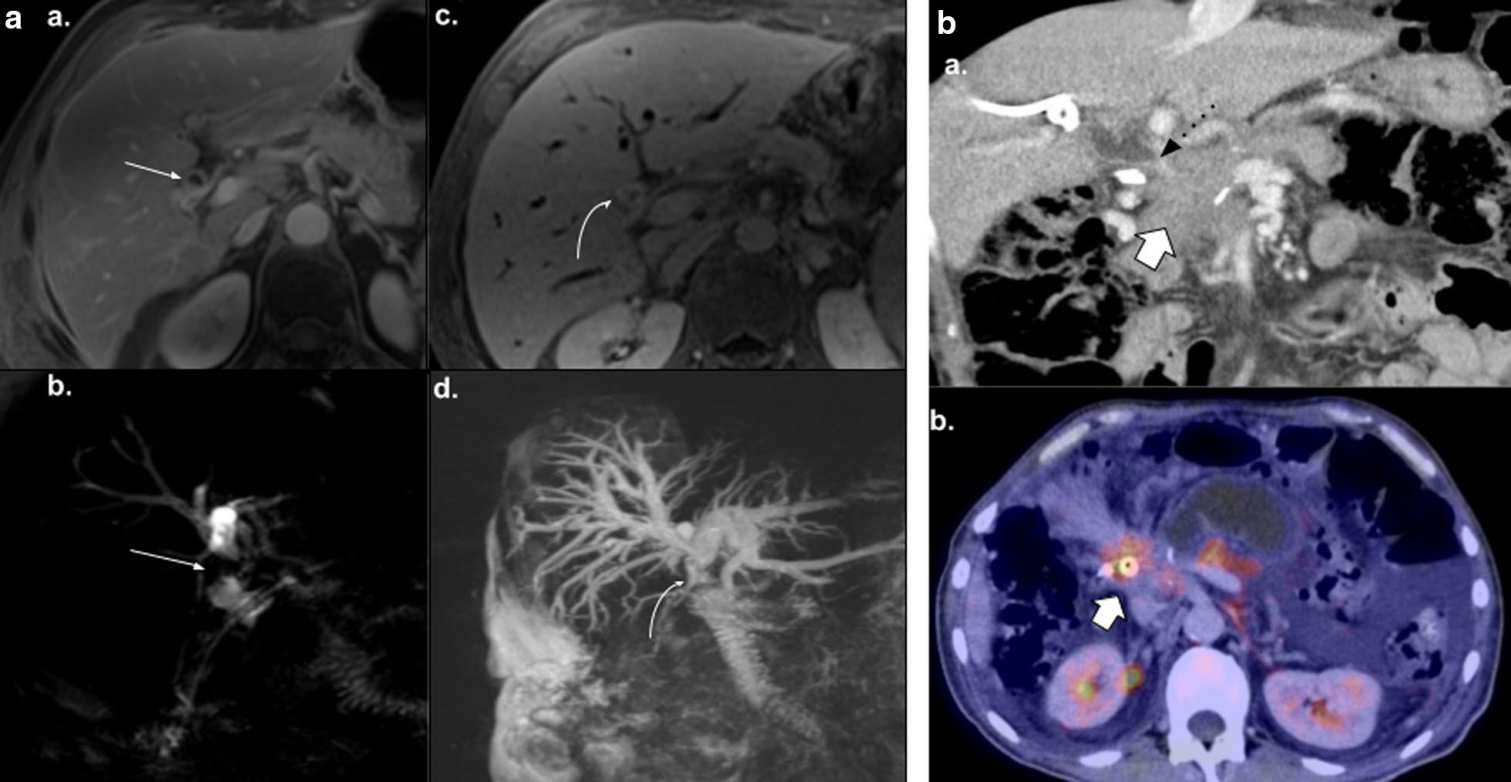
Hepaticojejunostomy stenosis. Two different patients (a) 67-year-old male patient with pancreaticoduodenectomy due to cholangiocarcinoma. All images below to normal postoperative control MRI. (a, b) one month after surgery (c, d) 7 months control. Note the caliber reduction in hepaticojejunostomy due to thickness of hepatic bile duct (curved arrows). Intraoperative cholangiography confirmed total hepaticojejunostomy stenosis (not shown) (b) 59-year-old male patient with abdominal discomfort and unexplained weight loss two years after pancreaticoduodenectomy owing to adenocarcinoma. CT scan denotes abrupt intrahepatic biliary stenosis (dotted arrow) due to ill-defined mass (big arrow). PET/CT fusion confirms local tumor recurrence as causal of stenosis
